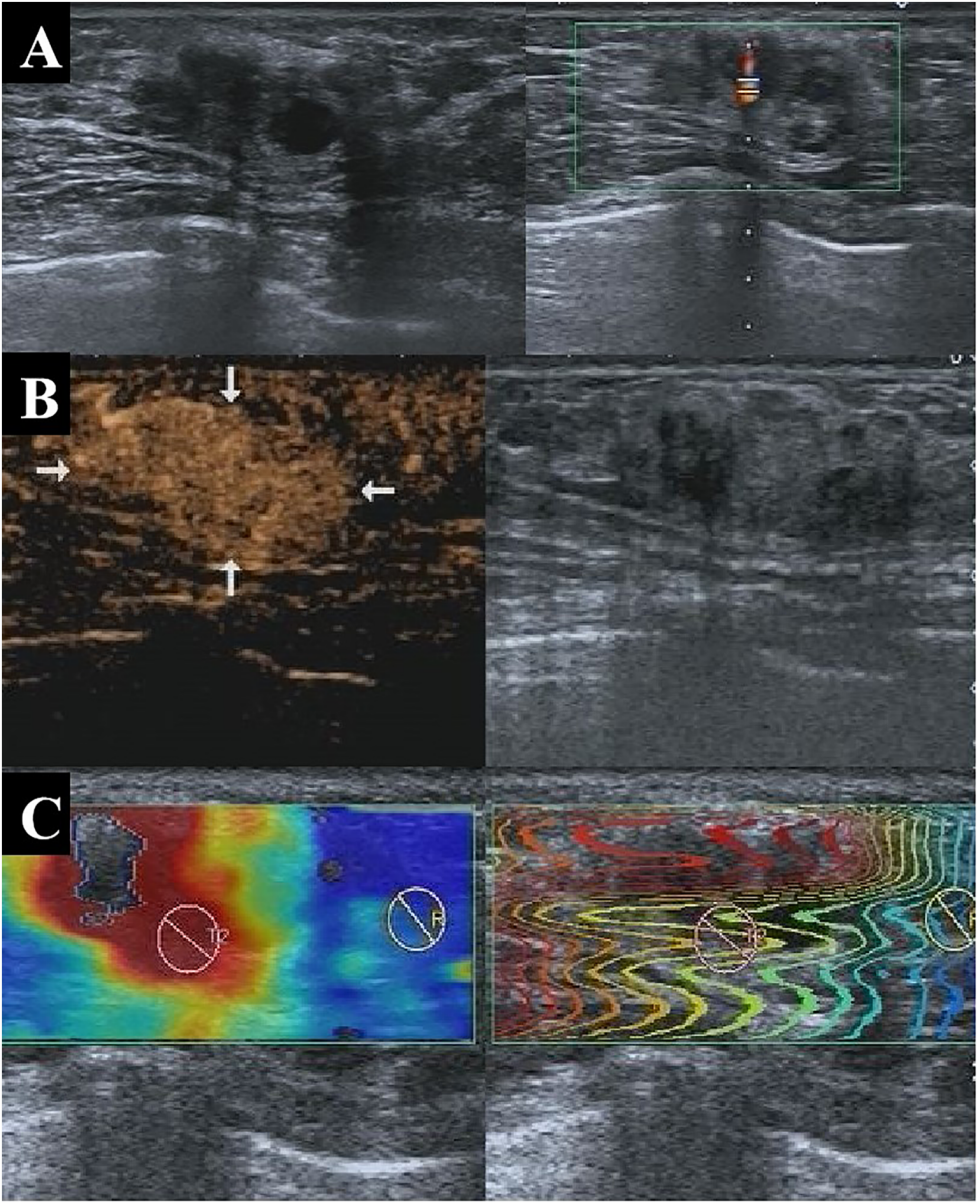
A 47-year-old patient with pathologically confirmed invasive ductal carcinoma showing an isoechoic solid nodule at 1 o’clock in the left breast with well-defined margins and a regular shape on conventional US (A), indicating a benign lesion with a BI-RADS 3 score. CEUS showed early hyperenhancement, enhanced area enlargement, contrast agent retention, clear margins, and irregular shape (B), indicating a malignant lesion with a BI-RADS 4b score. SWE also indicated that the lesion was malignant (C). The nodule was assessed as malignant by the multi-parameter predictive model. US, ultrasonography; CEUS, contrast-enhanced ultrasonography; BI-RADS, Breast Imaging Reporting and Data System; SWE, shear wave elastography.
